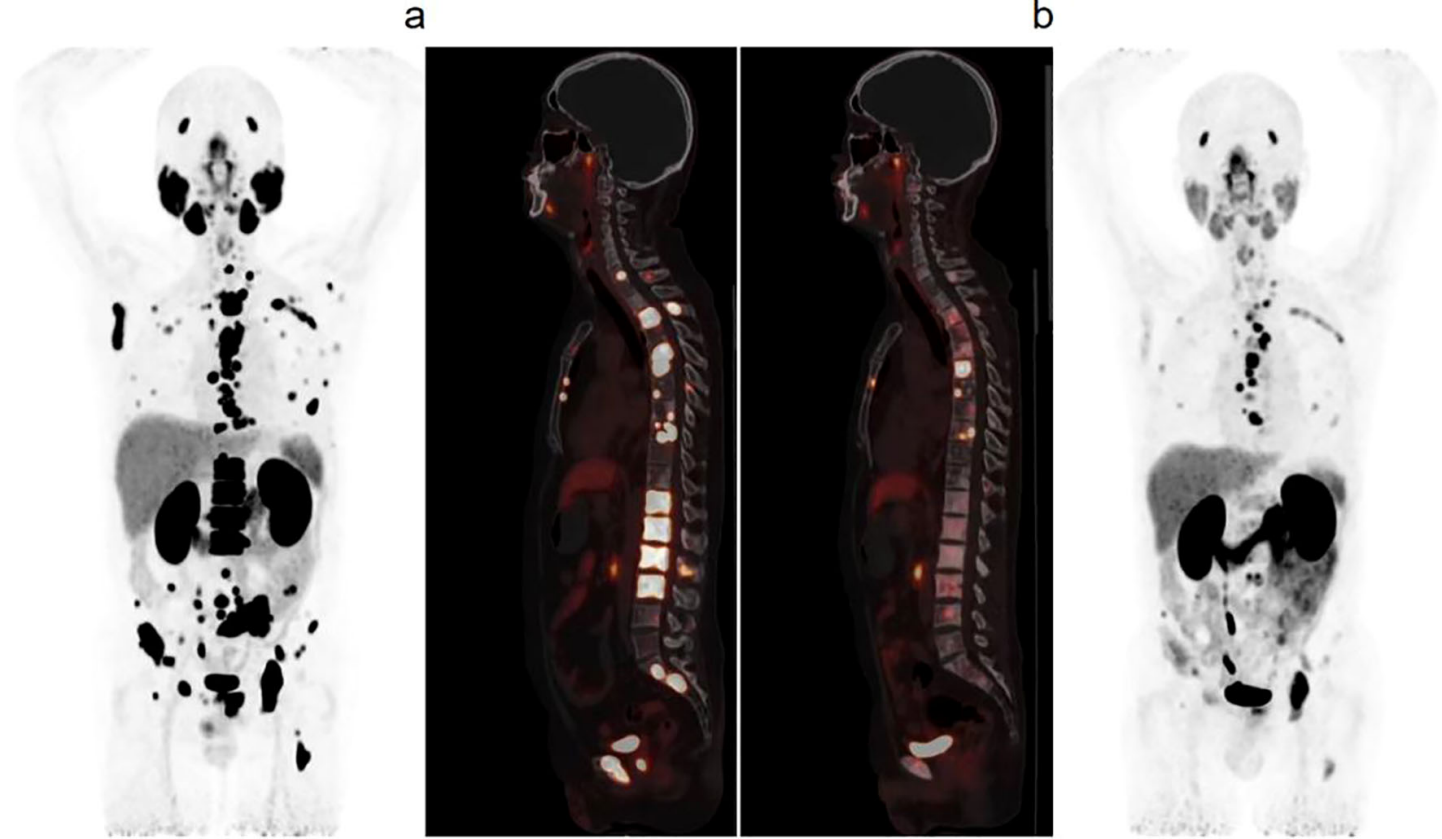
After 3 cycles of ^225^Ac-PSMA-617 treatment, the patient’s PSA decreased from 601.326 ng/ml to 7.486ng/ml. Compared to the baseline, ^68^Ga-PSMA-11 PET/CT images showed a significant decrease in tracer uptake in multiple metastases throughout the body after treatment (a, b).
